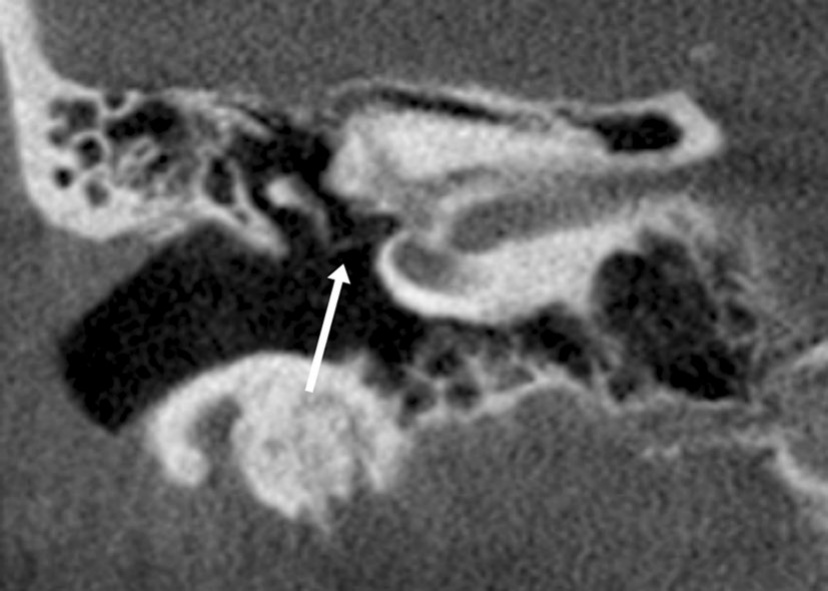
Example images in the coronal plane taken with HR CBCT demonstrate the capabilities and limitations of off-focus images for imaging the fine ossicle structures of the incus and the stapes
